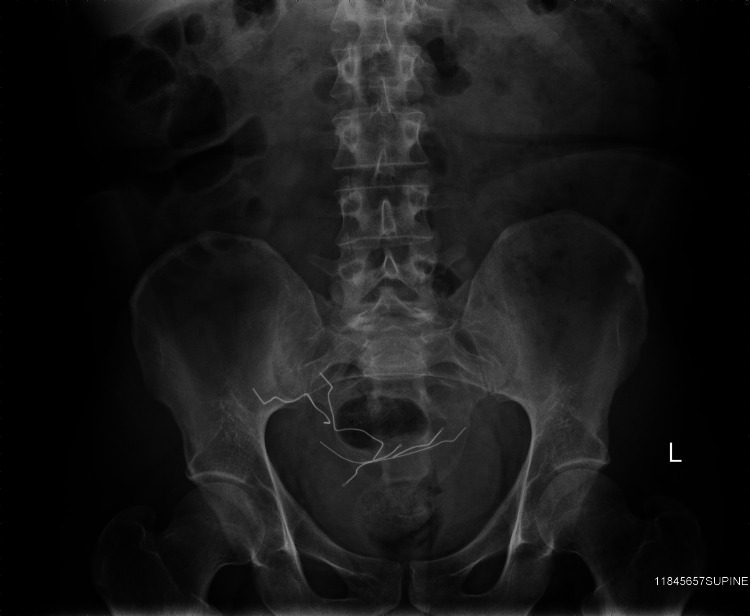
Abdominal X-ray with paperclips embedded in the pelvic floor.
